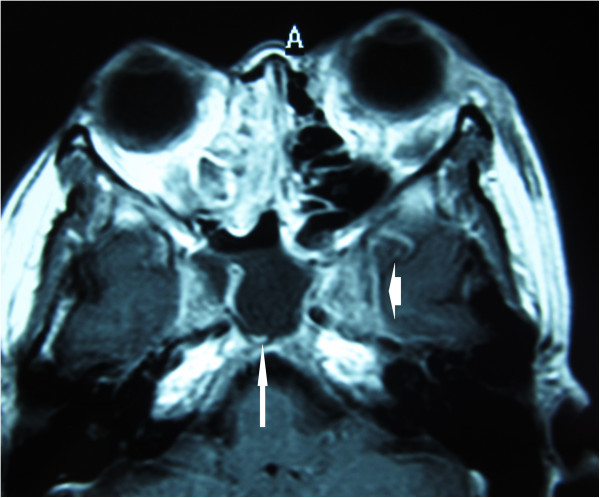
Contrast-enhanced MRI. Contrast-enhanced T1 MRI showed enhancement of the left cavernous sinus with some defects.
